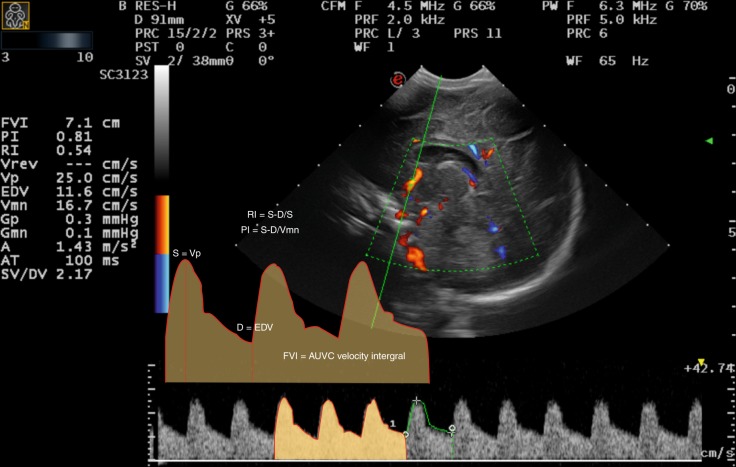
GMH/IVH: Doppler systematic review: arterial flow indices. AUVC area under the velocity curve, FVI flow velocity integral, Vmn mean velocity = MV in the text.
